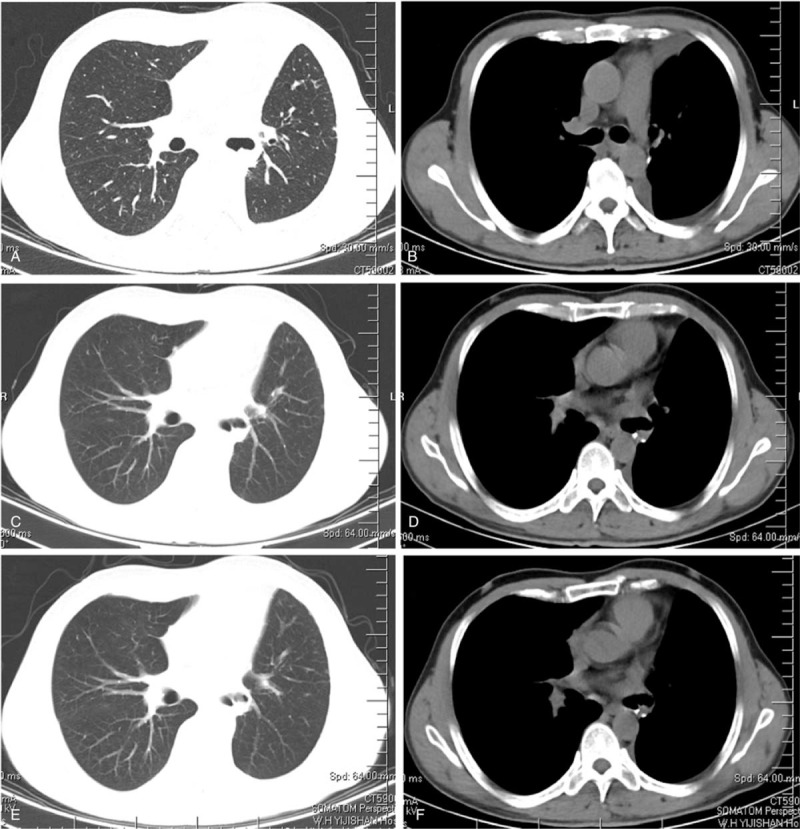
Postsurgery follow-up chest CT scans. A, B: The first follow-up chest CT after surgery was taken on June 24, 2020. C, D: The second follow-up postoperative chest CT scan was taken on September 2, 2020. E, F: The third follow-up postoperative chest CT scan was taken on November 4, 2020.
